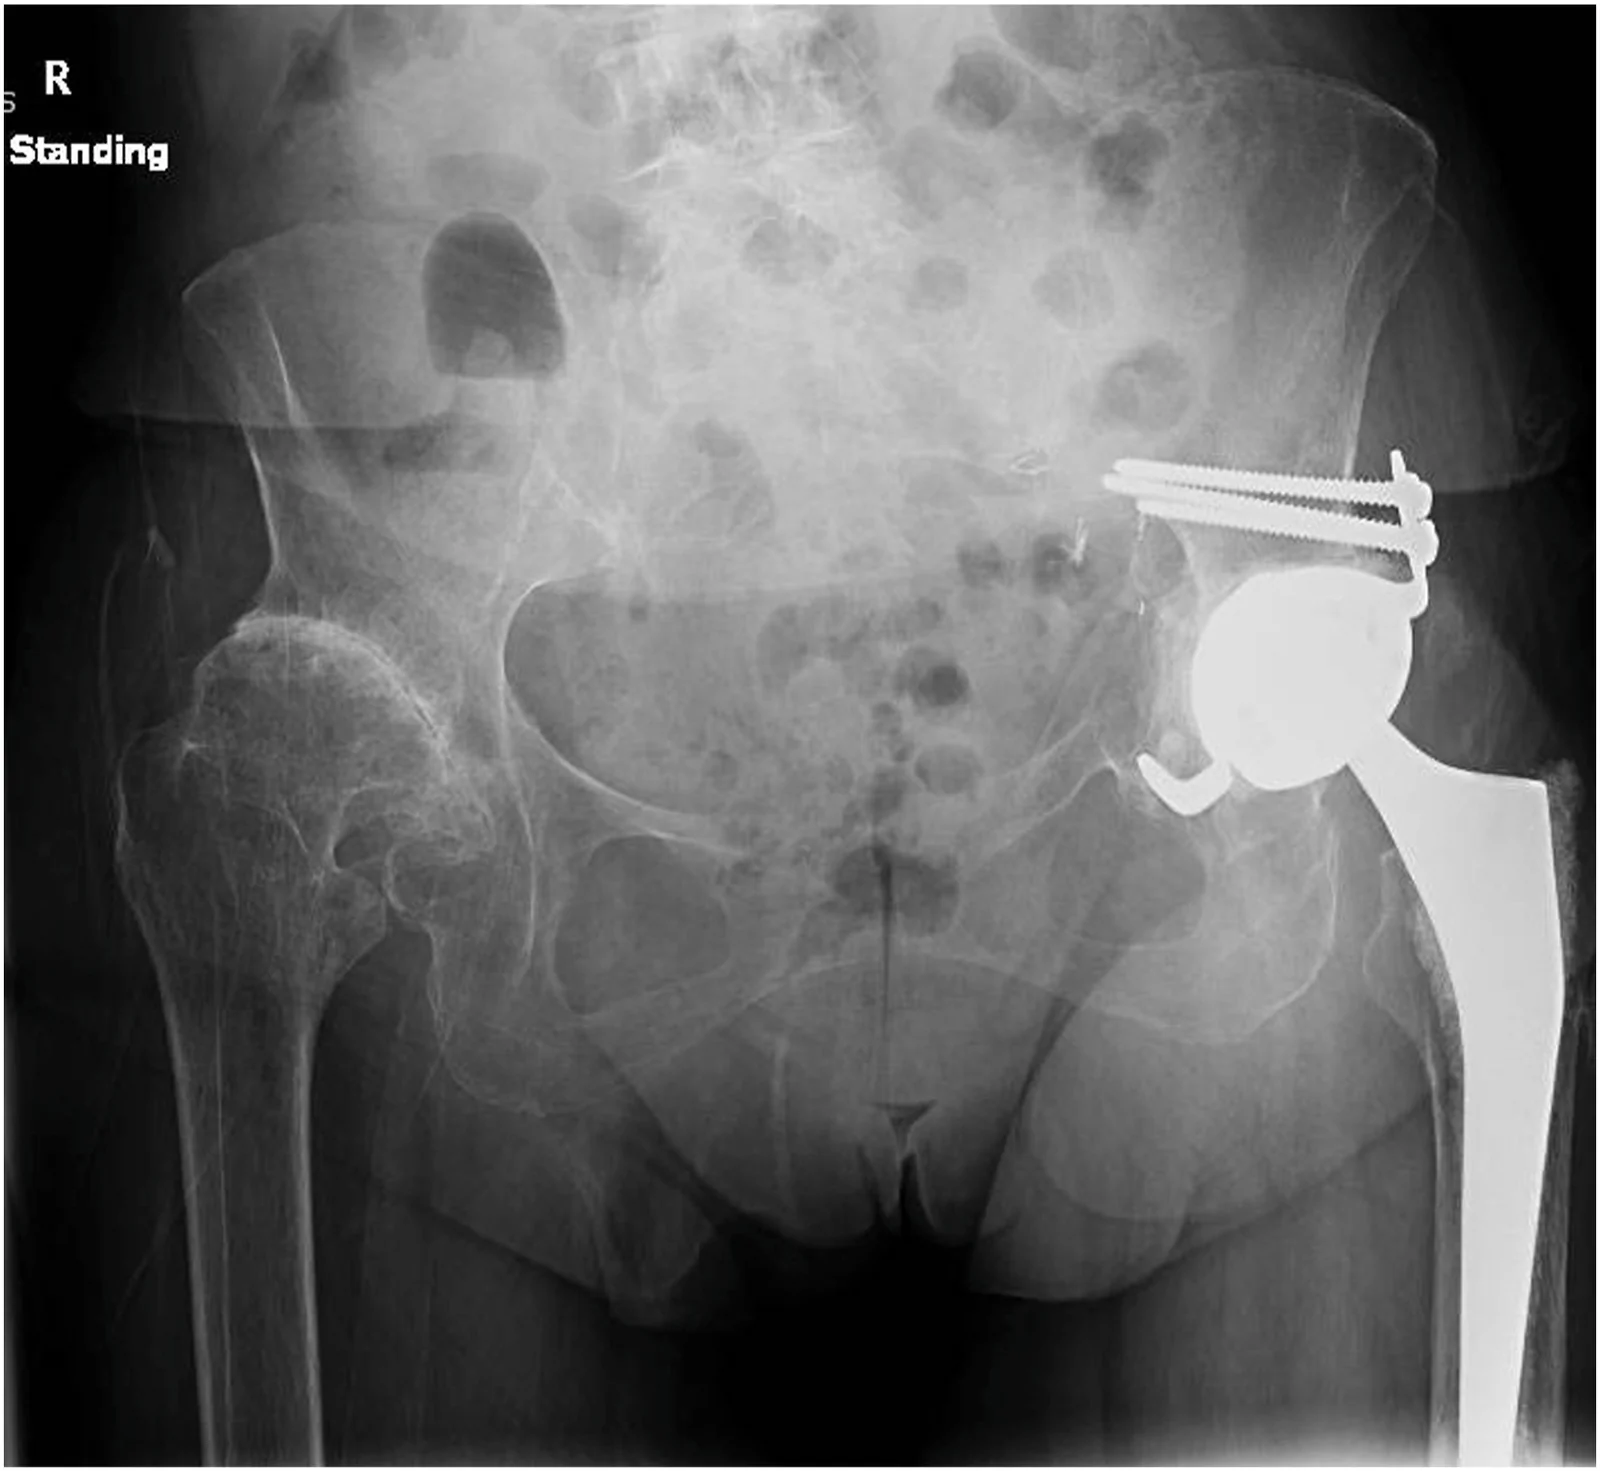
Radiographs 2.5 years postsurgery.
